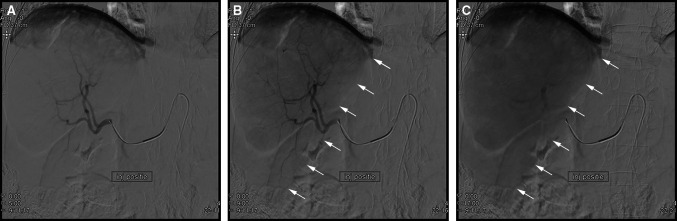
Example of how the C-arm CT scan delay was determined on power injection DSA in the right hepatic artery. A Start of the DSA run. Only vascular contrast enhancement is visible. B Midway the DSA run. Parenchymal contrast enhancement of the right liver lobe (*white arrows* indicate the border of the right liver lobe) is starting to show. C At the end of the DSA run, maximal parenchymal contrast enhancement is reached. The time between the first and last run is used as delay for the C-arm CT scan
